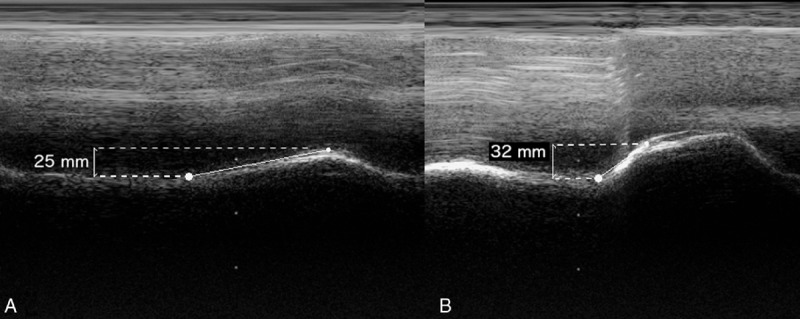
Left hemidiaphragmatic motion recorded in M-mode sonography during (a) quiet breathing and (b) voluntary sniffing.
